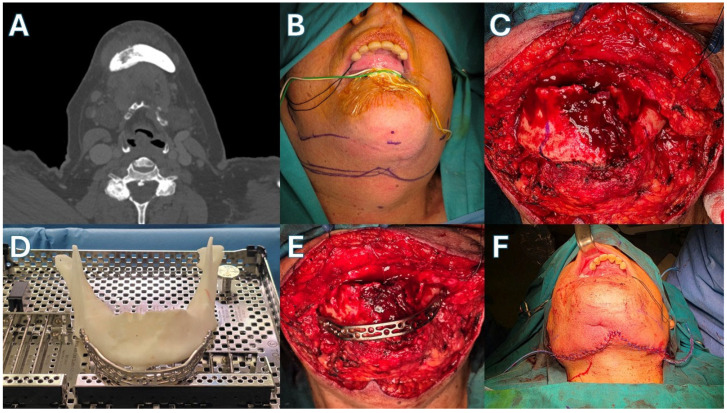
Case 2. (A) Pre-op CT showing necrotic mandibular lesion. (B) Incision design and marginal mandibular nerve neuromonitoring cables in place. (C) Exposed mandible with lesion. (D) Pre-bended titanium plate using a 3D model. (E) The plate in place. (F) Final incision closure.
